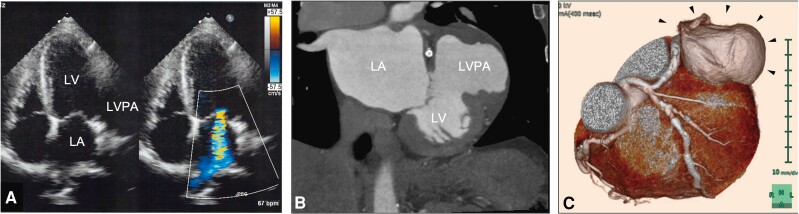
Cardiac imaging studies. (*A*) A transthoracic echocardiogram showing a huge aneurysm involving the lateral wall of the left ventricle with high-grade mitral valve regurgitation. (*B*) Cardiac computed tomography angiography revealing a contrast-filled outpouching on the lateral wall of the left ventricle. (*C*) A three-dimensional multiplanar reconstruction of the left ventricular pseudoaneurysm (black arrows). LA, left atrium; LV, left ventricle; LVPA, left ventricular pseudoaneurysm.
